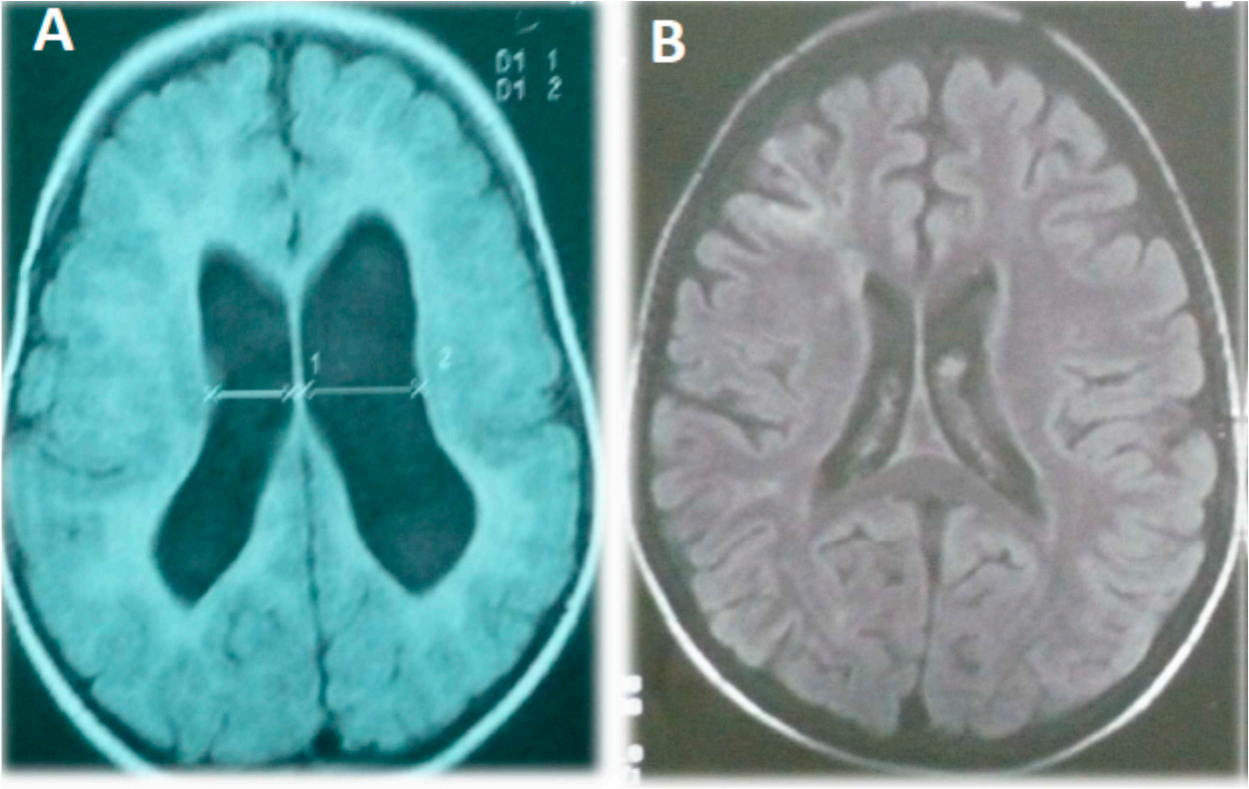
Magnetic resonance image of patient 2 (A) and 3 (B) showing an increase in lateral cerebral ventricle volume, diffuse enlargement of the cortical spaces, and cortical atrophy.
